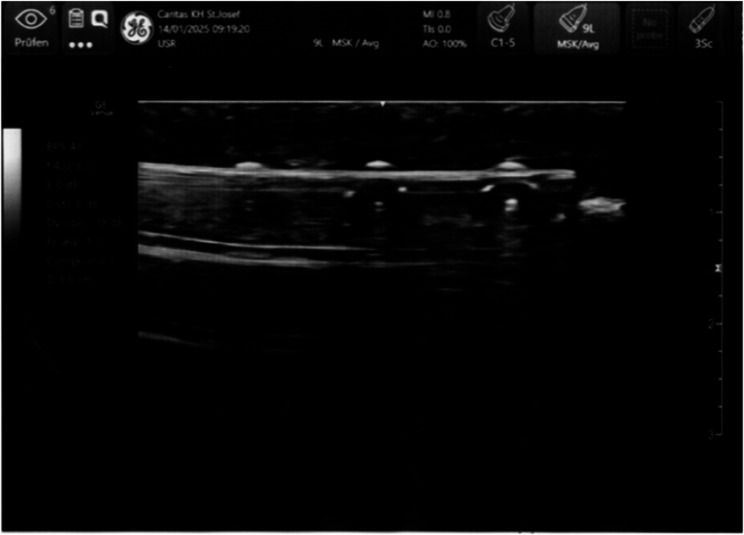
Sonographic image of a plate with screws in the longitudinal plane. The individual screws, the plate and the bone can be clearly distinguished. The image corresponds to a 59-year-old female patient (BMI 22.8) with ankle fracture (type Weber B) and syndesmotic injury
